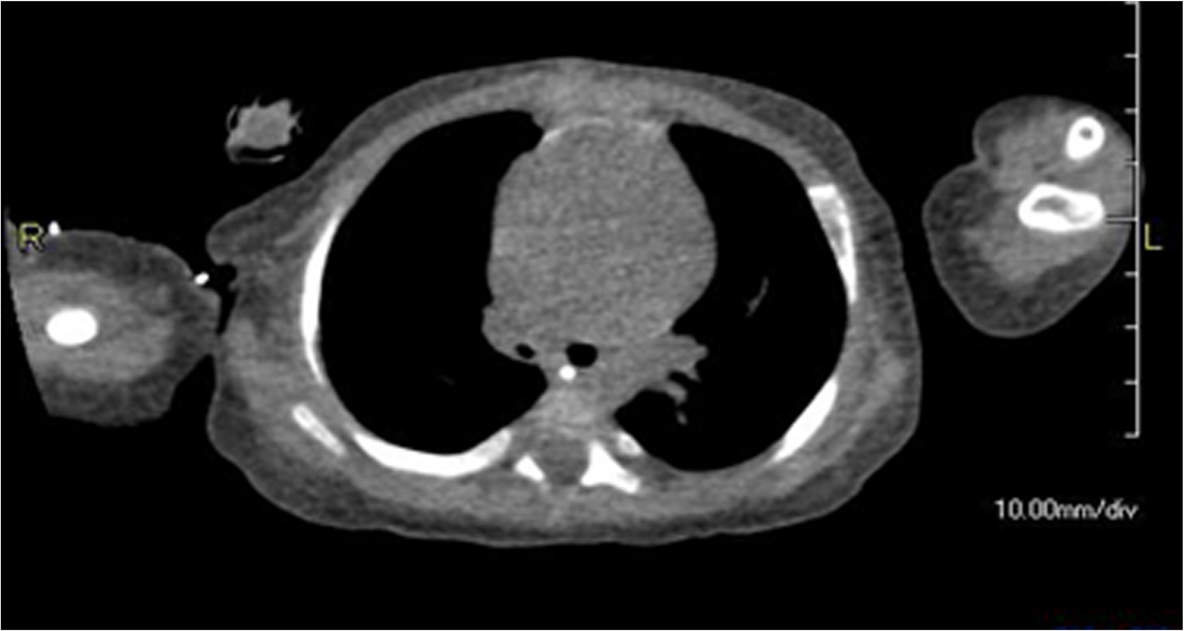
Chest computed tomography on day 50. Right bronchus was narrower than the left.
